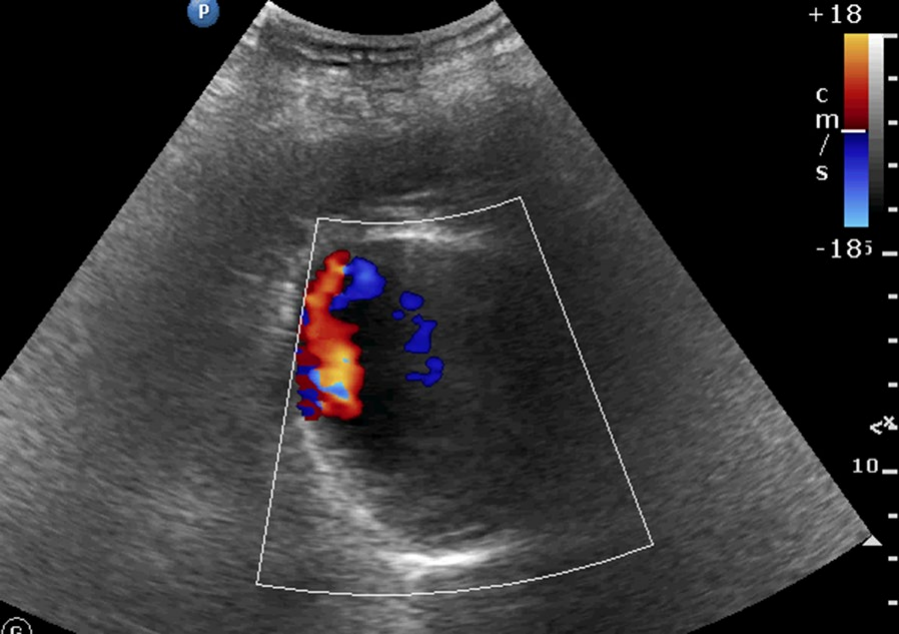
POCUS color Doppler scan of left upper quadrant in a patient presenting an epigastric pain. See the strong Doppler signal
